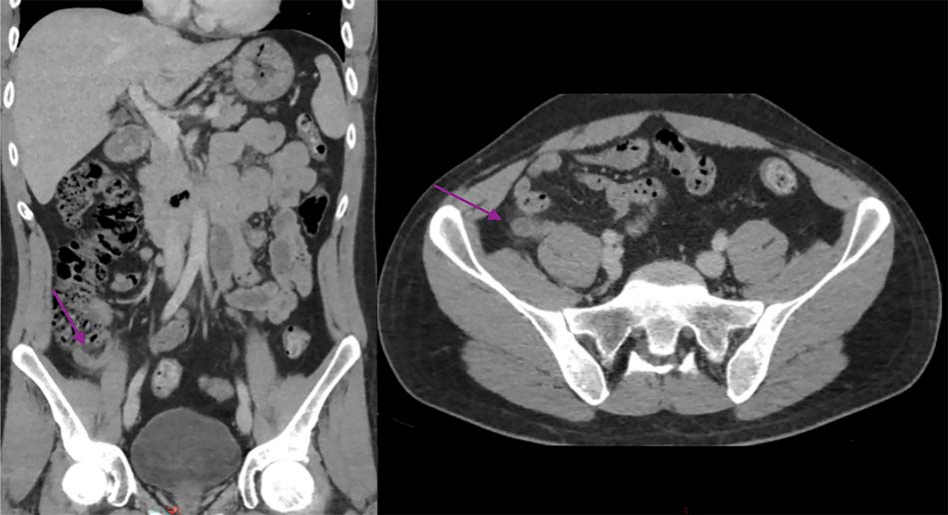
Abdominal CT scan showing a dilated and thickened appendix with periappendiceal fat stranding (arrow); coronal and axial view.
